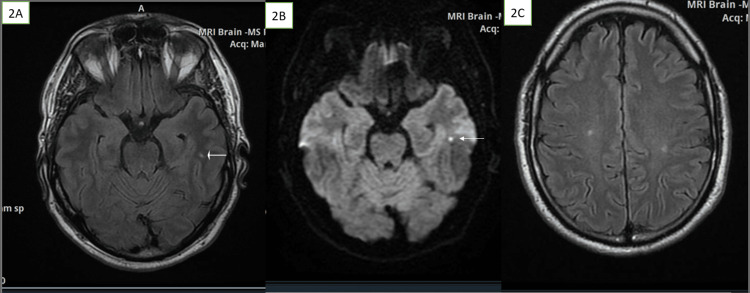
Brain MRI in March 2022 after the appearance of new symptoms (2A) A small new lesion in the left temporal lobe demonstrating a hyperintense signal on the FLAIR sequence (arrow). (2B) The same lesion showing diffusion restriction on the diffusion-weighted sequence. (2C) Some of the old lesions showing from August 2020, showing gliotic features on the FLAIR sequence FLAIR: fluid-attenuated inversion recovery
